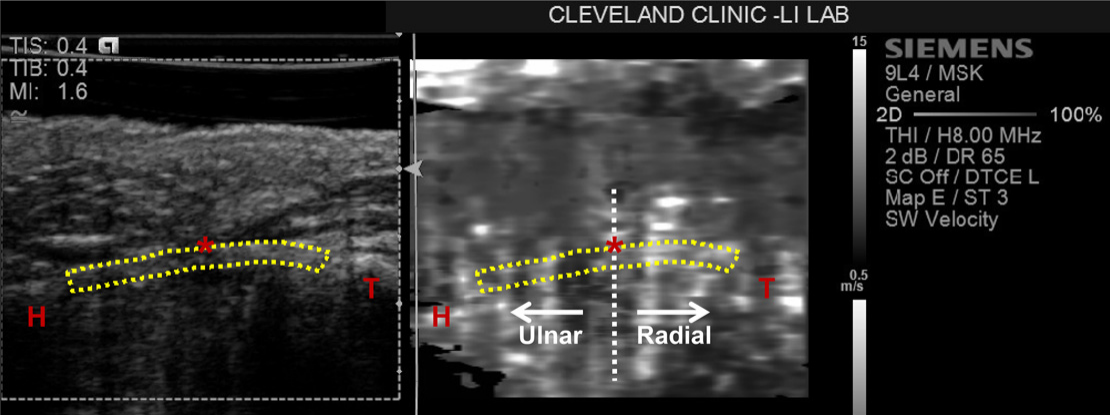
A representative ultrasound image with the TCL outlined on both the B-mode side (left) and ARFI side (right). The hamate (H), trapezium (T), and thenar muscles’ ulnar point (TUP,*) are identified. The vertical line at the TUP represents the location where the TCL was divided into radial and ulnar portions. Reprinted under a CC BY license, with permission from the Hand Research Laboratory at Cleveland Clinic, original copyright 2016.
